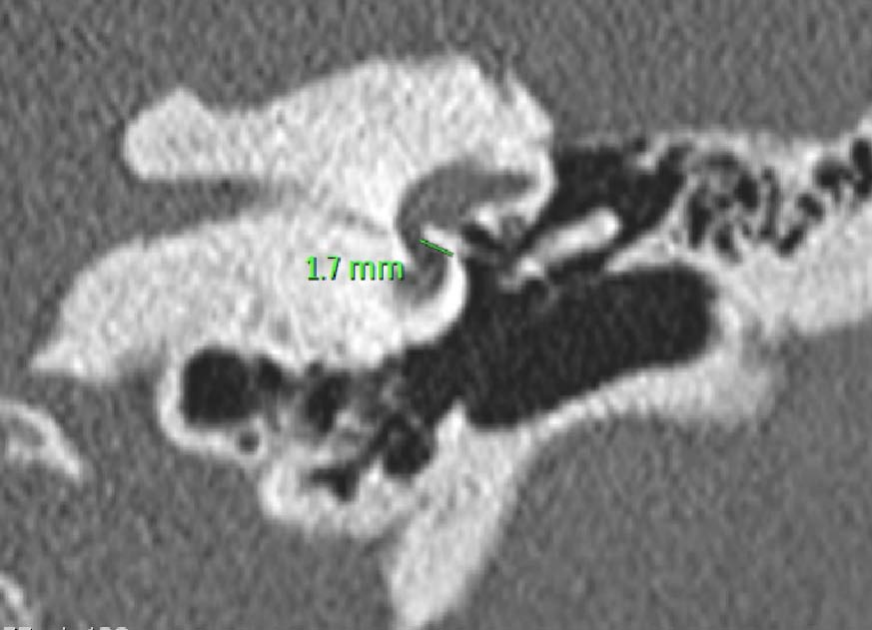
High-resolution computed tomographic (CT) scan showing a left stapes prosthesis. There appears to be an otospongiotic focus by the anterior lip of the stapes footplate. The platinum-Teflon prostheses appear to be extending deep into the vestibule.
